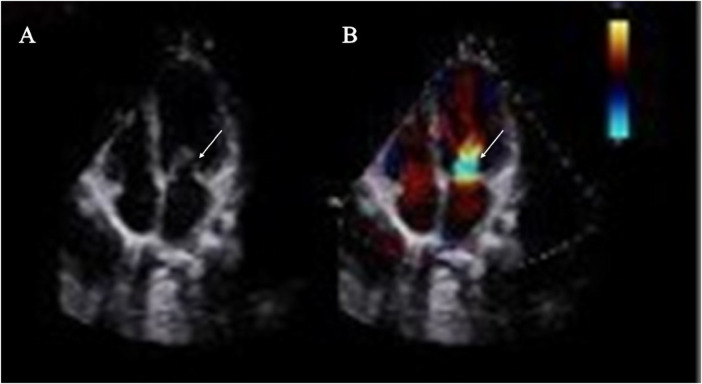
Transthoracic echocardiography. (A) Apical four-chamber two-dimensional view showing a membranous ridge above the mitral leaflets on the left atrial side (arrow), consistent with a supravalvular (supramitral) ring and associated left atrial enlargement. (B) Color Doppler imaging in the same view demonstrating aliasing and a high-velocity turbulent jet across the mitral inflow at the level of the supravalvular ring (arrow), corresponding to a peak transmitral velocity of 2.46 m/s and a mean pressure gradient of approximately 24 mmHg, with mild mitral regurgitation.
